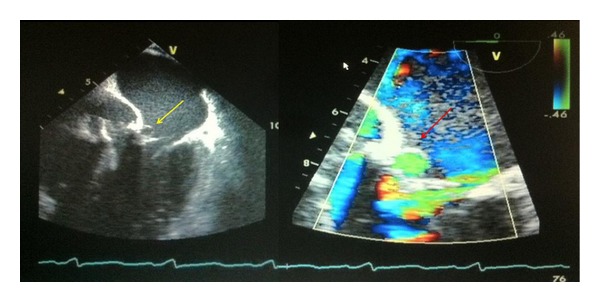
TEE depicting systolic ballooning of the aneurysmal segment of the P-MAIF (yellow arrow) and Doppler evidence of flow within the P-MAIF (red arrow).
